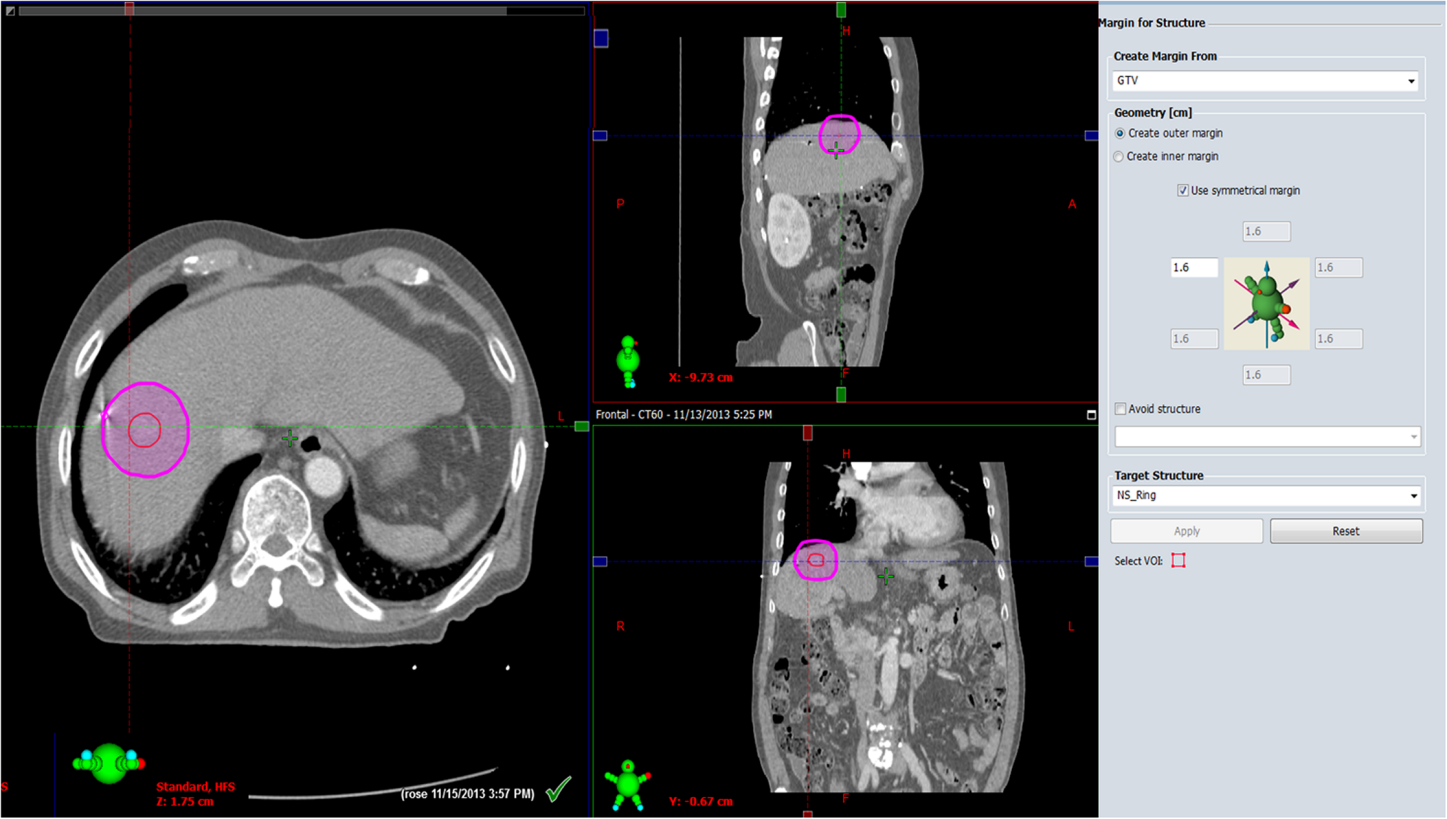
Calculating the distance semi-automatically between the tumor margin and a marker in 3-dimensional space creating a curved line parallel to the outer margin of the tumor using planning CT workstation. Firstly, draw a line along tumor margin. Second, create a curved line parallel to the outer margin of the tumor at marker site using workstation tool. And the distance between the tumor margin and a marker is automatically calculated as 1.6cm.
